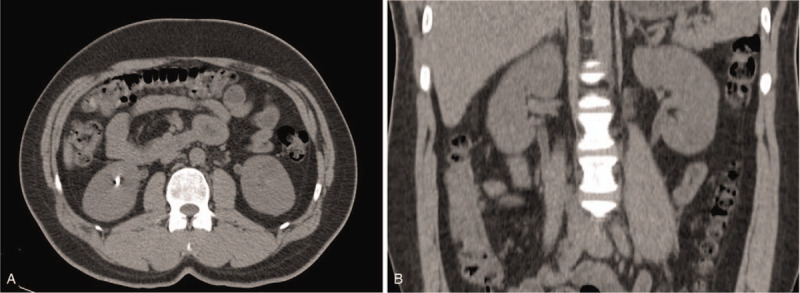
Computed tomography (CT) without intravenous contrast media shows complete resolution of the lesion at follow-up 3 months after discharge.
